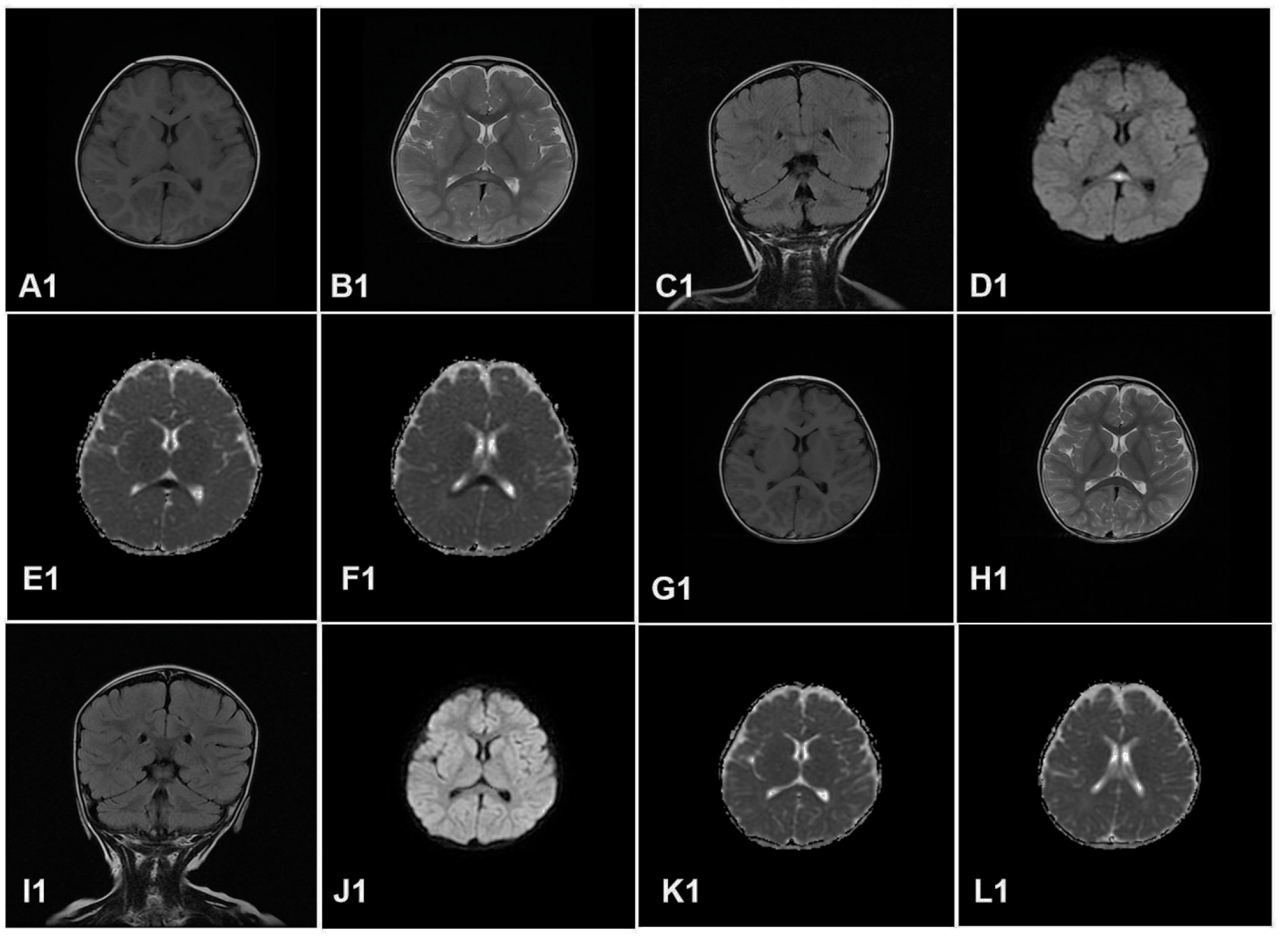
A case of RESLES type-1. Cranial MRI revealed an isolated lesion in the splenium of the corpus callosum on day 4. (A1–F1): slightly hypointense on T1WIs (A1); hyperintense signal on T2WIs (B1), FLAIR images (C1), and DWIs (D1); low value on apparent diffusion coefficient (ADC) maps (E1, F1). Cranial MRI revealed that all the lesions had disappeared completely on day 9 after treatment (G1–L1).
